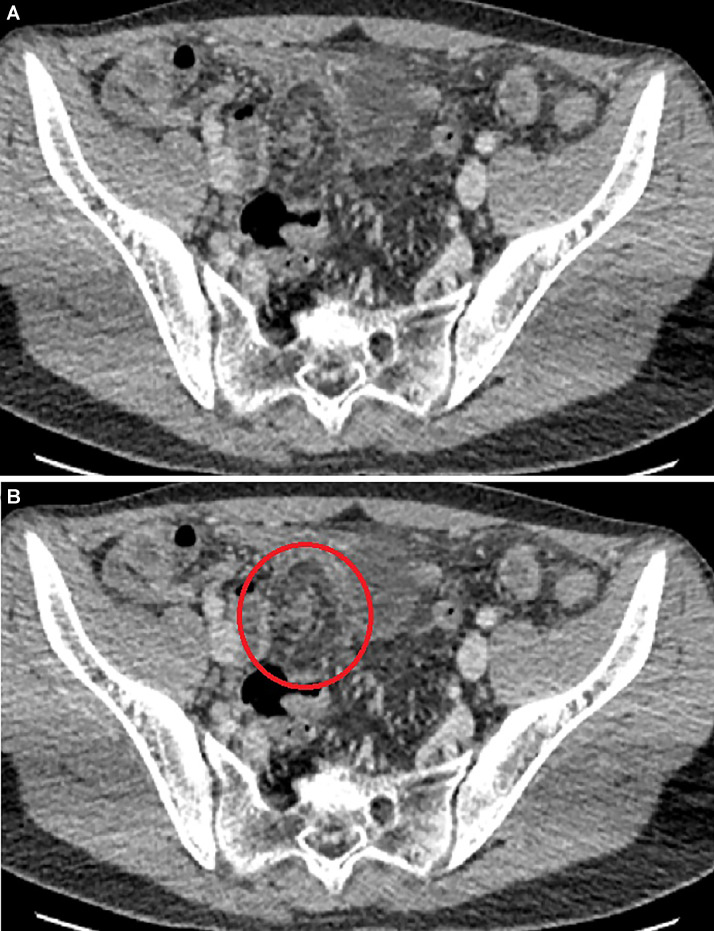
Axial contrast-enhanced CT view showing omental torsion. (A) The whirlpool sign is highlighted by the red circle (B).
